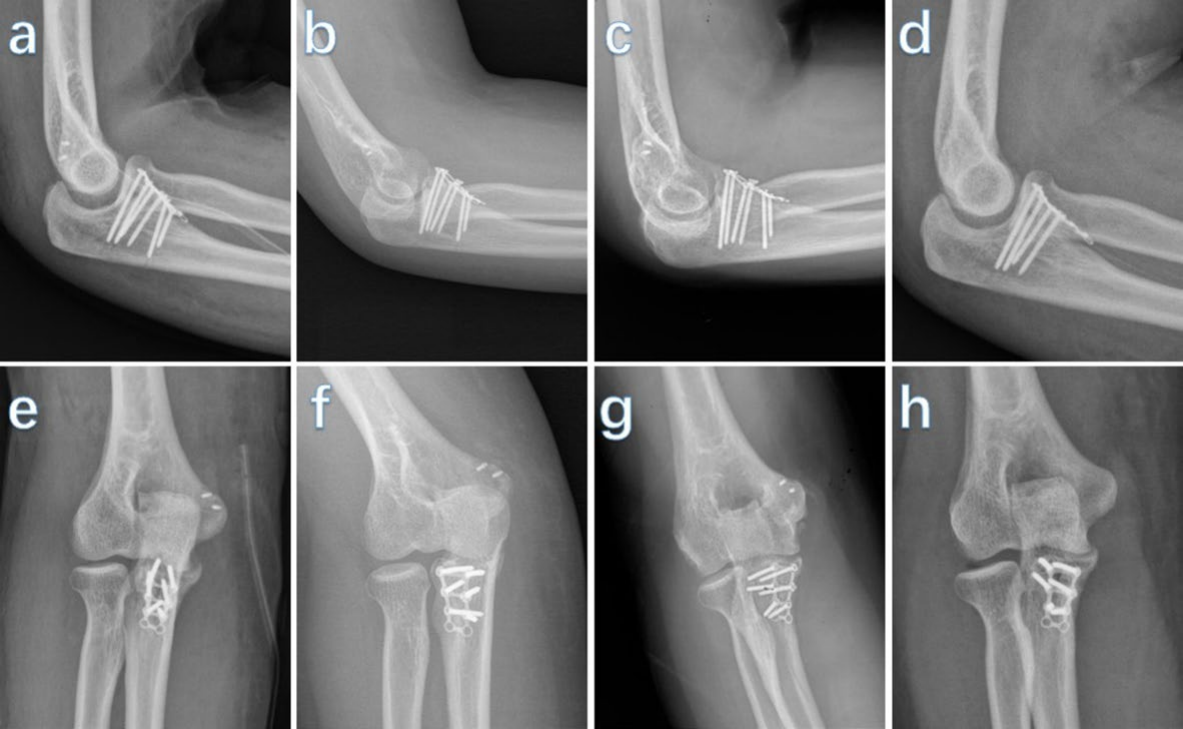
Radiographs obtained during follow-up. (a, e) After 4 weeks. (b, f) After three months. (c, g) After 6 months. (d, h) 12 months after the operation
